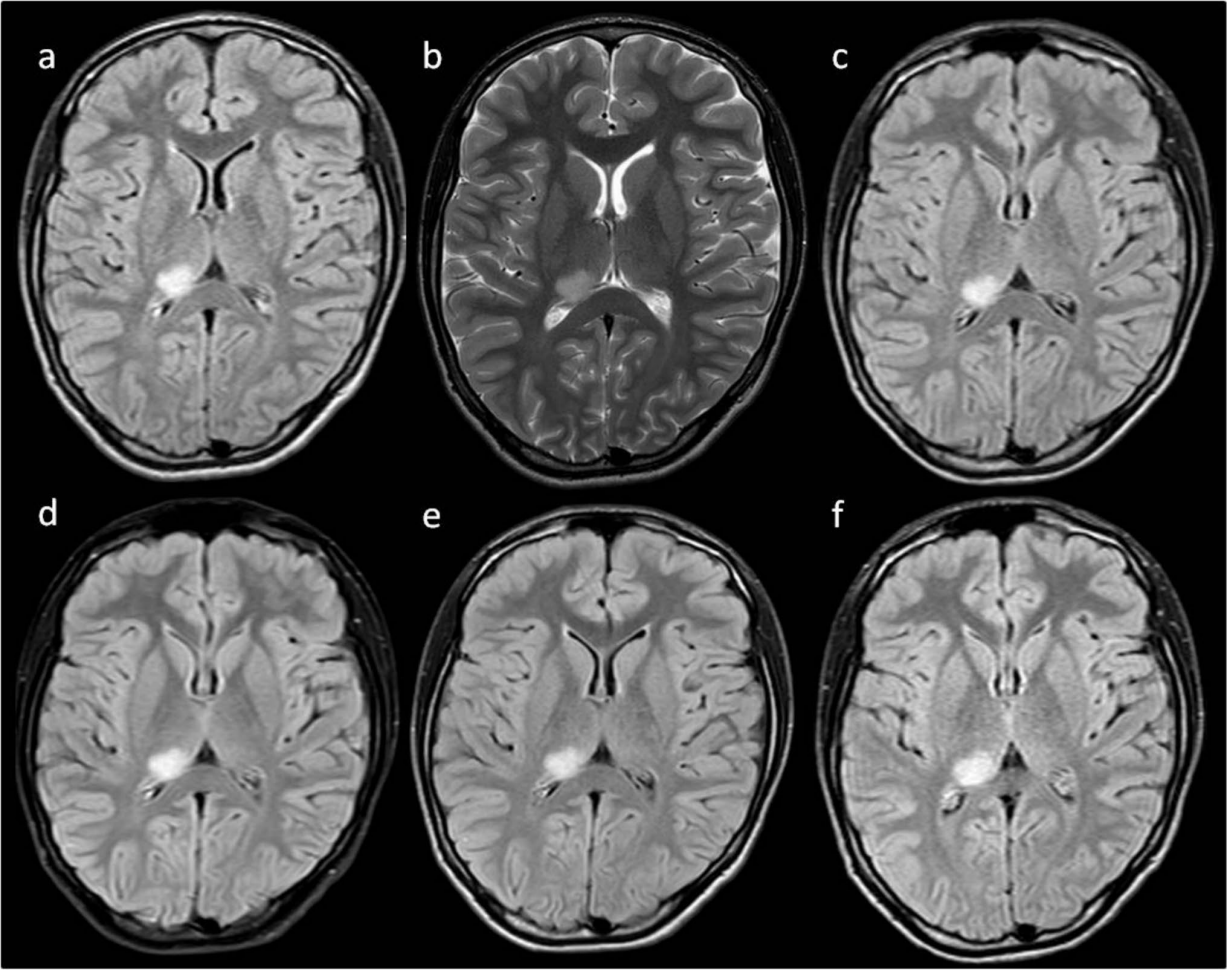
Example of a stable incidentaloma. A 15-year-old boy was examined for headaches. A hypersignal lesion on FLAIR (a, c, d, e, f) and T2 (b) images in the right posterior thalamus was diagnosed and remained stable for the duration of a follow-up of 29 months (c-f)
